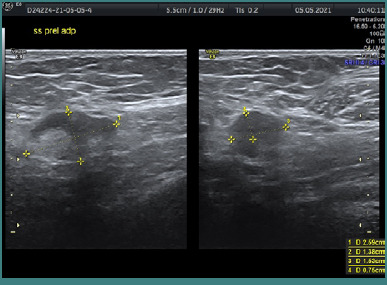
Ultrasound-guided punctured axillary adenopathy
